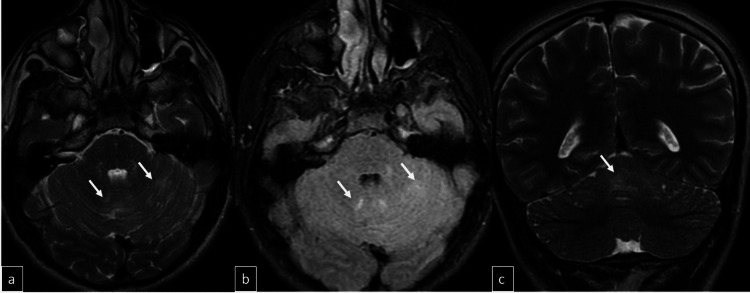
Patchy areas of increased signal intensity with folial effacement consistent with edema are observed in the cerebellum on axial T2-weighted (a), FLAIR (b), and coronal T2-weighted (c) images (arrows). FLAIR: fluid-attenuated inversion recovery
